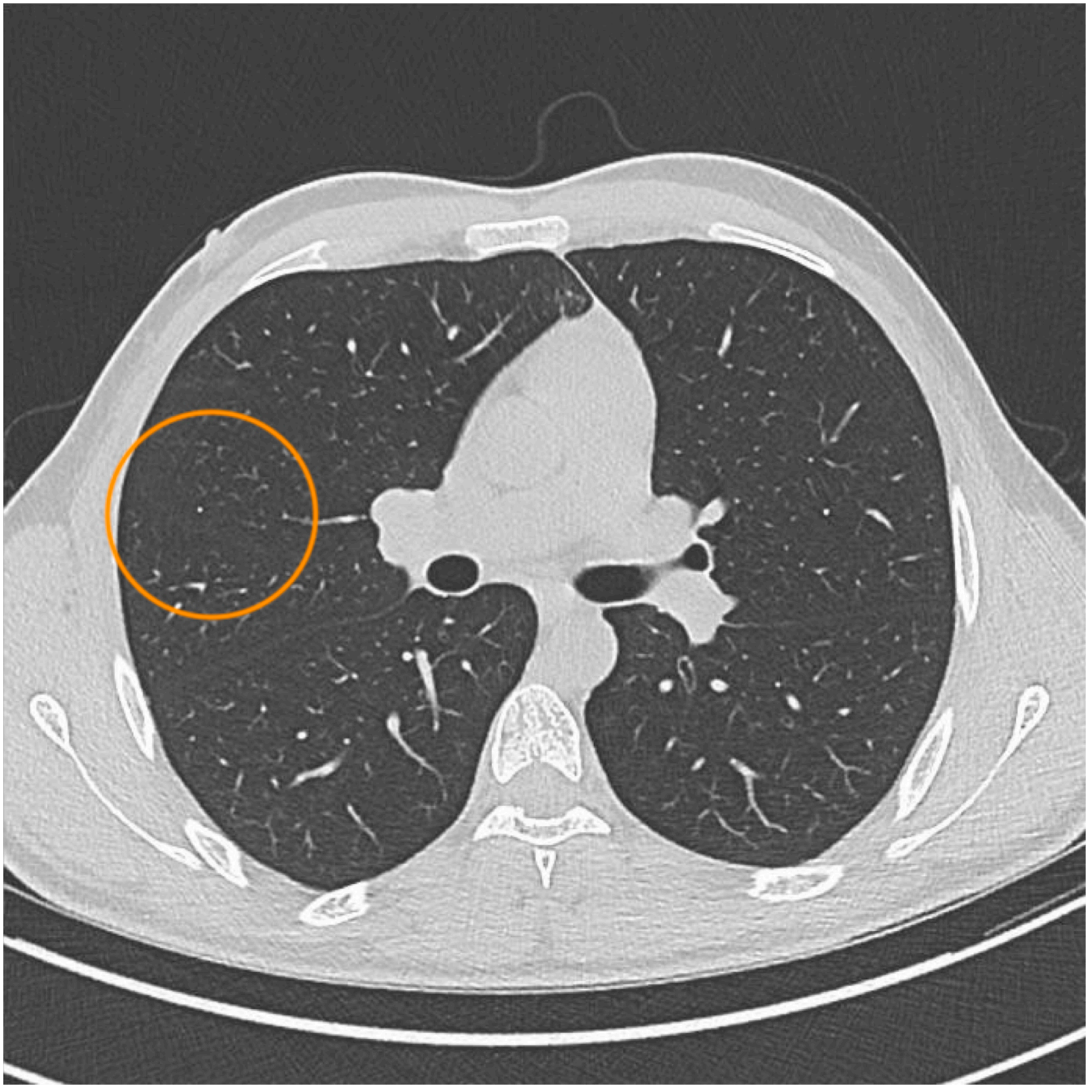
CT thorax at the time of treatment initiation showing persisting small granuloma and diminished ground glass opacities. CT = computed tomography.
